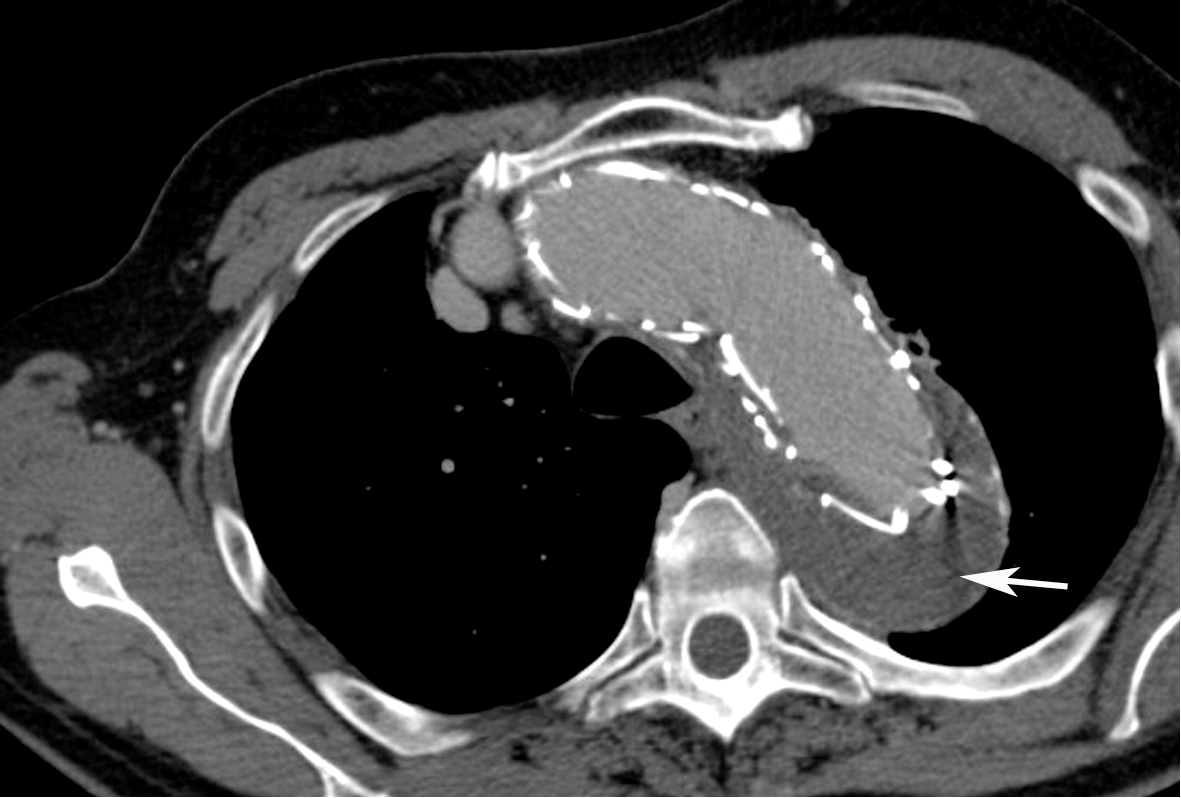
Control CT-scan 3 years after stent-graft extension shows a stable thoracic aortic diameter of 50 mm without contrast opacification of the excluded false lumen, both in the early arterial and in the late venous phase.
